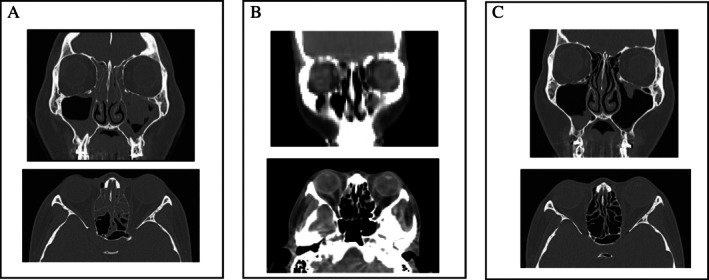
Sinus CT image taken on March 24, 2018, prior to mepolizumab administration, showing findings of severe sinusitis, mainly in the ethmoidal sinus region. (A) PET‐CT image taken on April 24, 2020, more than 1 year after starting mepolizumab, showing marked improvement in sinusitis. (B) Sinus CT image taken on August 17, 2022, 2 months after starting dupilumab, showing no change compared to B and no evidence of recurrent sinusitis.
